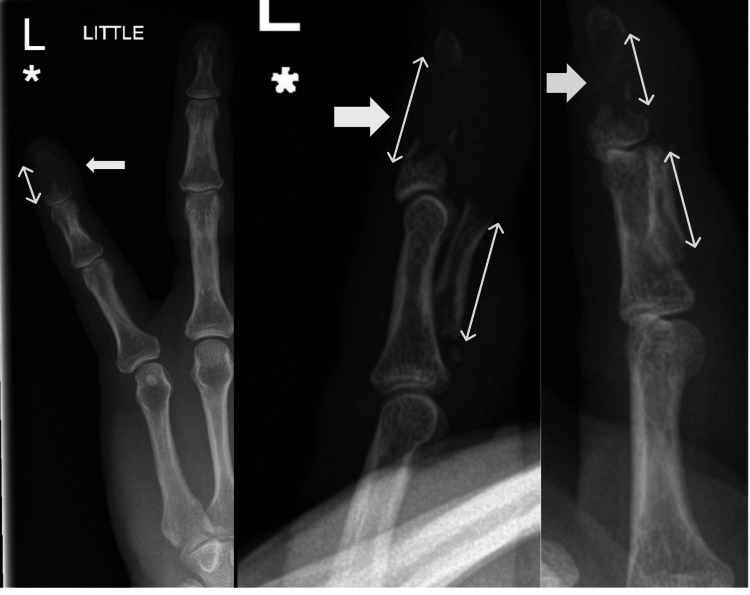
Pre-operative antero-posterior, oblique, and lateral radiographs showing large extra-articular distal phalanx fragment
